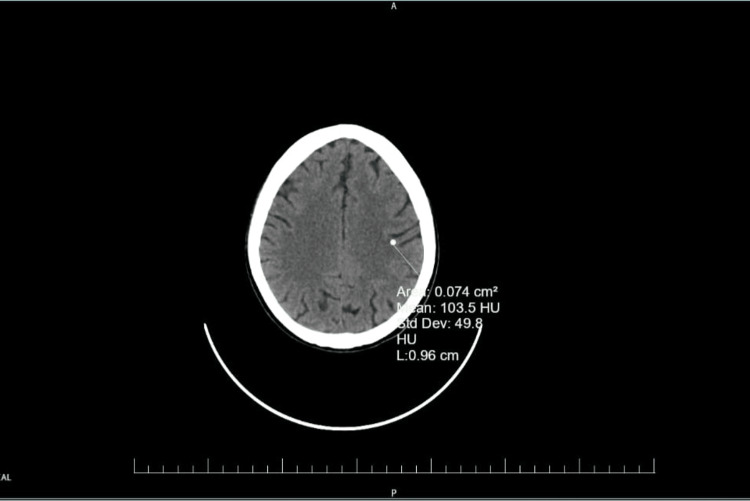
Axial image of non-contrast computed tomography at the level of the parietal region of the brain showing a calcified lesion in the left parietal lobe, consistent with old neurocysticercosis
